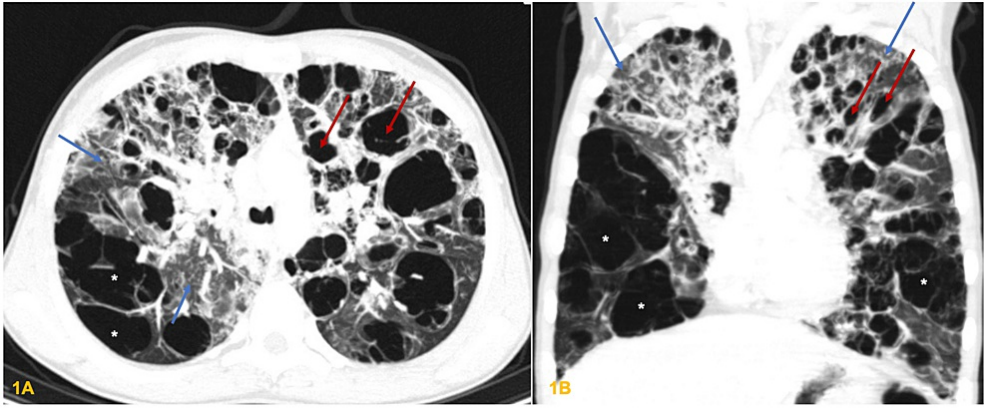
CT scans showed centrilobular and paraseptal emphysema (stars), extensive varicoid and cystic bronchiectasis (red arrows), mucoid impaction, and ground glass opacification areas (blue arrows). CT: computed tomography
